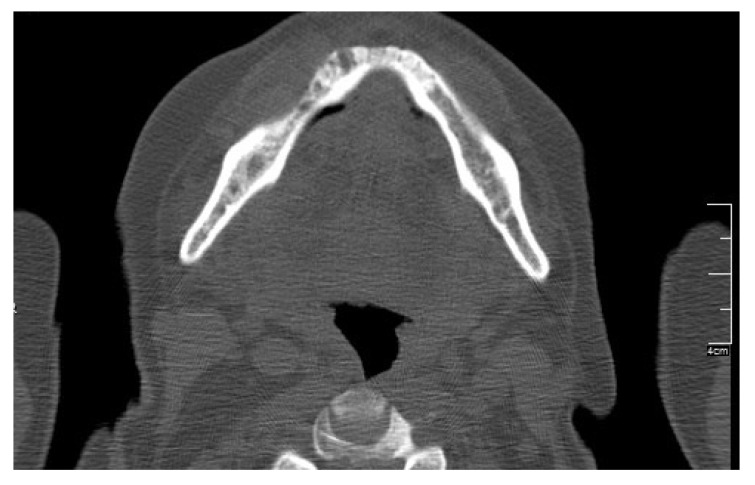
Computed tomography showing mandibular medication-related osteonecrosis of the jaw.
